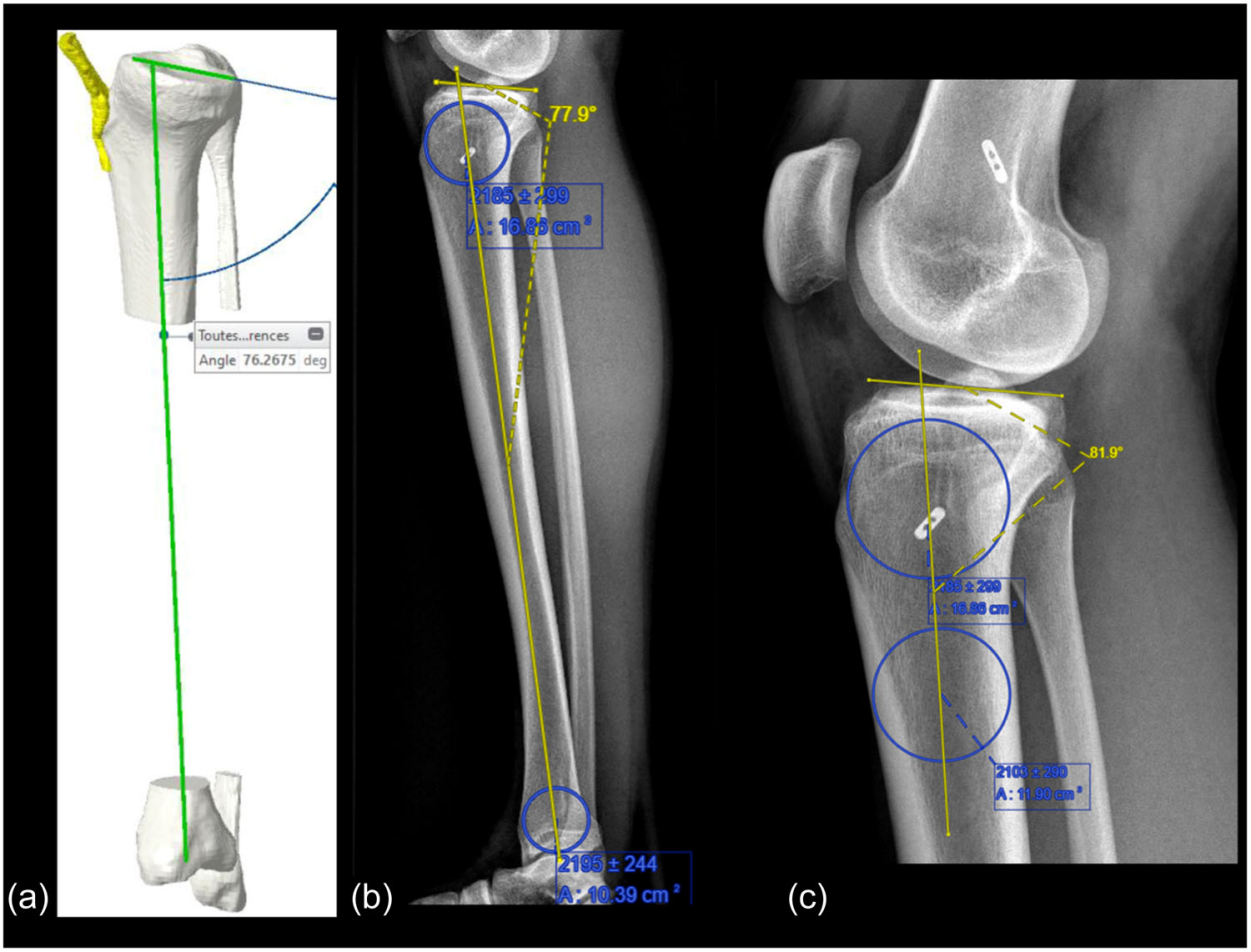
Demonstrates. (a) MPTS measurements on the 3D reconstructed CT images; (b) MPTS measurements on the long lateral radiograph (full‐length tibia); (c) MPTS measurement on the short lateral knee radiographs. 3D, three‐dimensional; CT, computed tomography; MPTS, medial posterior tibial slope.
